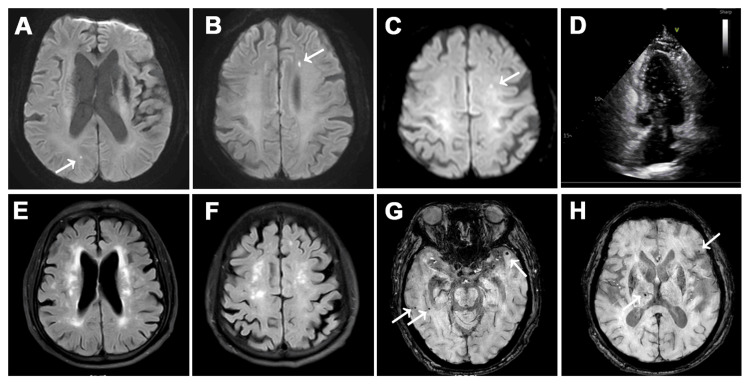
Follow-up MRI and right heart contrast echocardiography (A-C) Brain MRI (DWI) shows acute lacunar infarcts in the left centrum semiovale (A) (October 8, 2024) and a new infarct in the right occipital (B) and left frontal lobes (C) (February 18, 2025) (arrows). (D) Right heart contrast echocardiography reveals immediate entry of more than 30 microbubbles into the left atrium and ventricle following right-sided chamber filling, indicating a large right-to-left shunt consistent with PFO. (E-F) MRI FLAIR depicts extensive periventricular (E) and subcortical (F) white matter hyperintensities (Fazekas grade 3). (G-H) MRI SWI displays multiple cerebral microbleeds in the basal ganglia and cortex (arrows). DWI: Diffusion-weighted imaging; PFO: Patent foramen ovale; FLAIR: Fluid-attenuated inversion recovery; SWI: Susceptibility-weighted imaging
